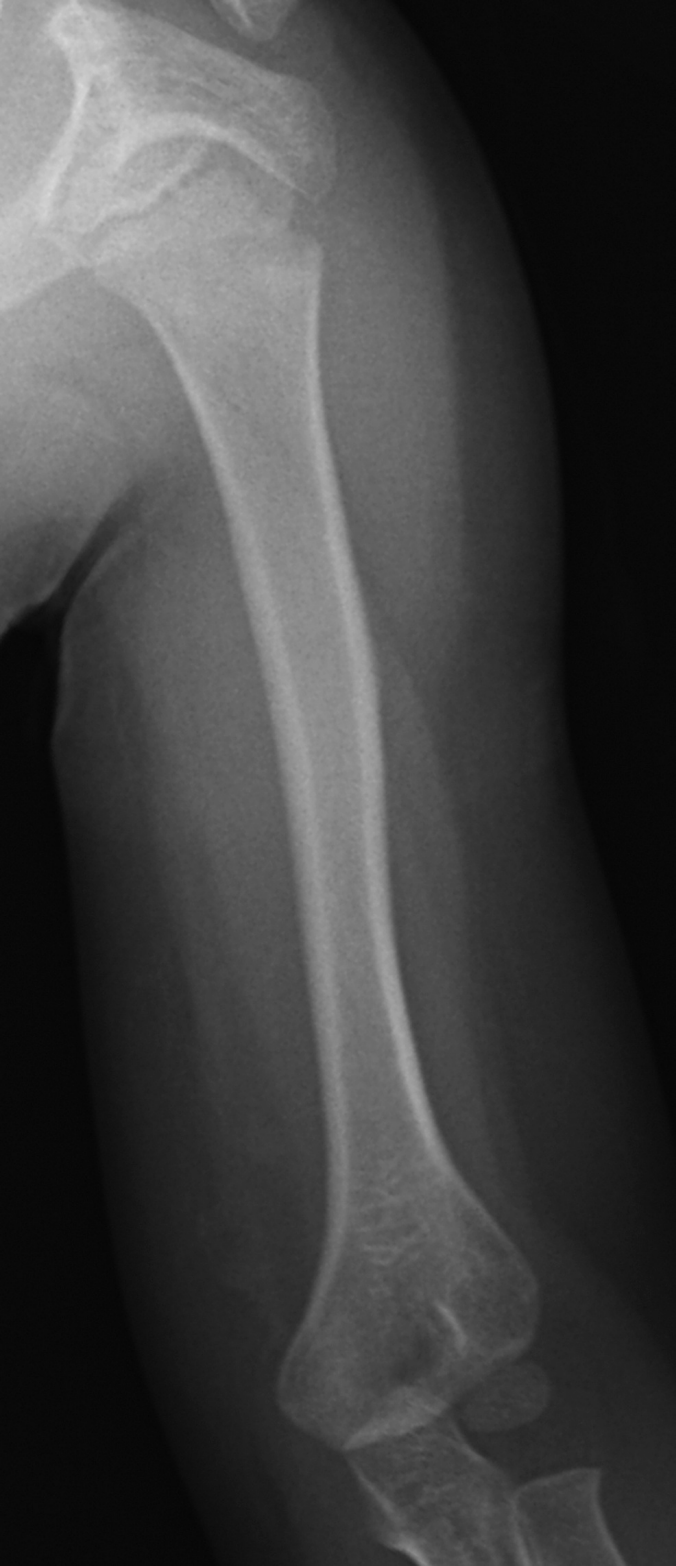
Anteroposterior radiograph of the left humerus taken 36 months after diagnosis showing complete resolution of the osteolytic bone lesion.
